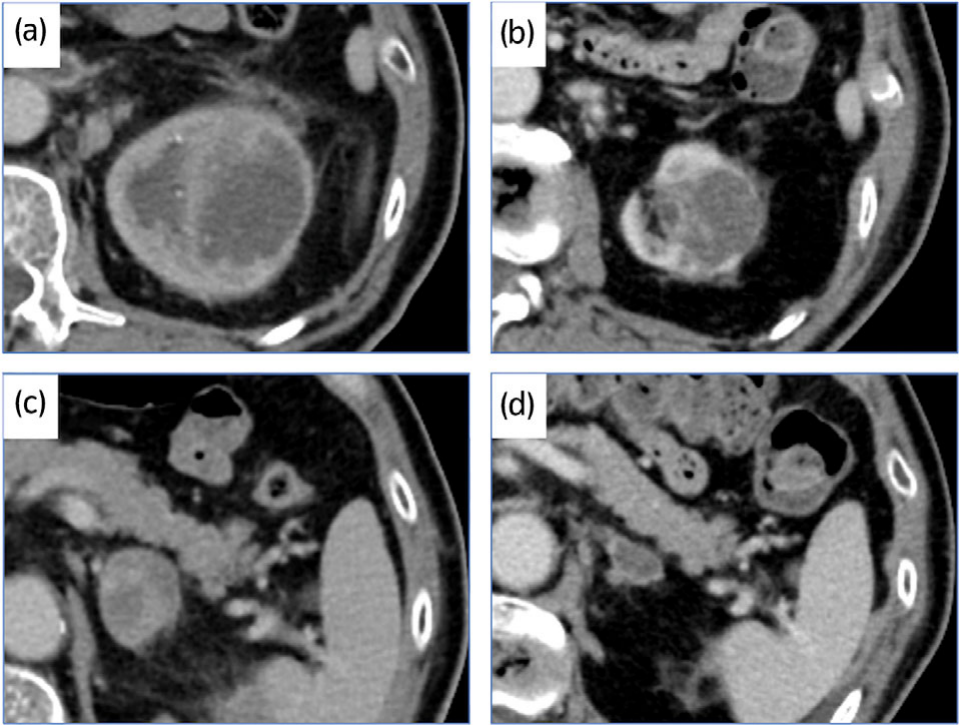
CT images in case 2. There is a left renal mass on initial diagnosis (a) and after 14 cycles of pembrolizumab (b). In addition, there is left adrenal metastasis on initial diagnosis (c) and after 14 cycles (d).
